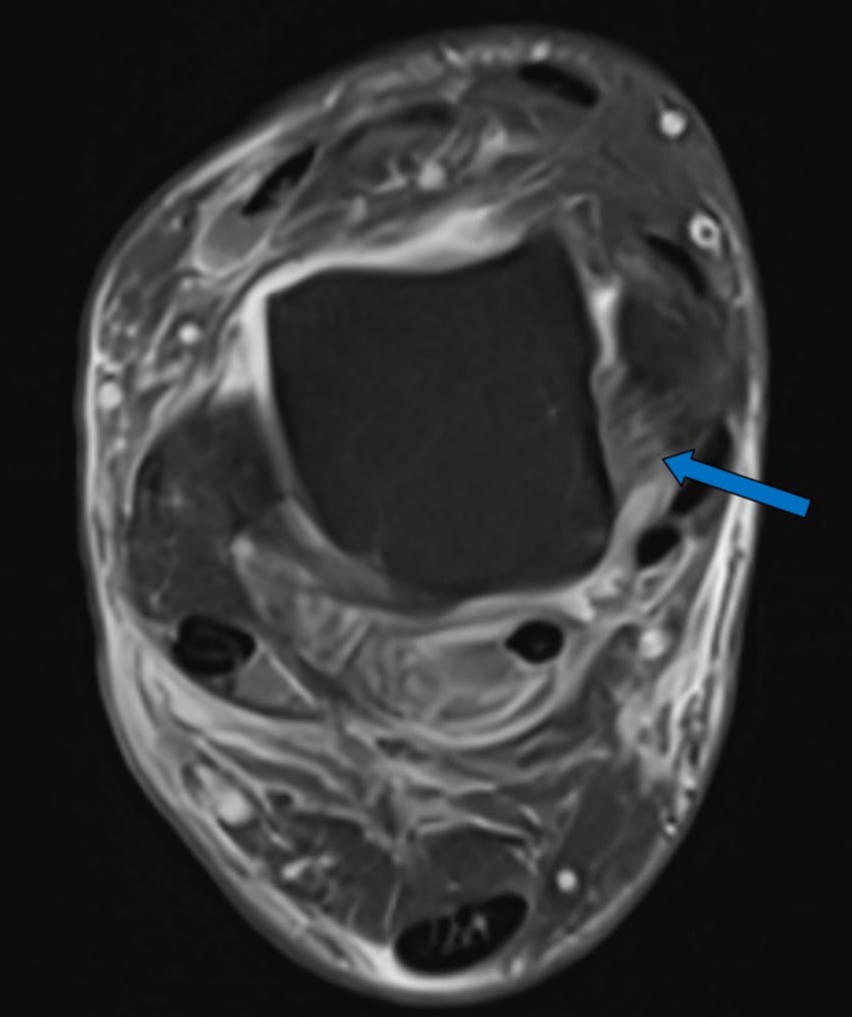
Magnetic resonance image (axial, proton density-weighted fast spin echo) showing marginal signs of edema in an otherwise intact dPTTL (arrow). dPTTL, deep posterior tibiotalar ligament.
